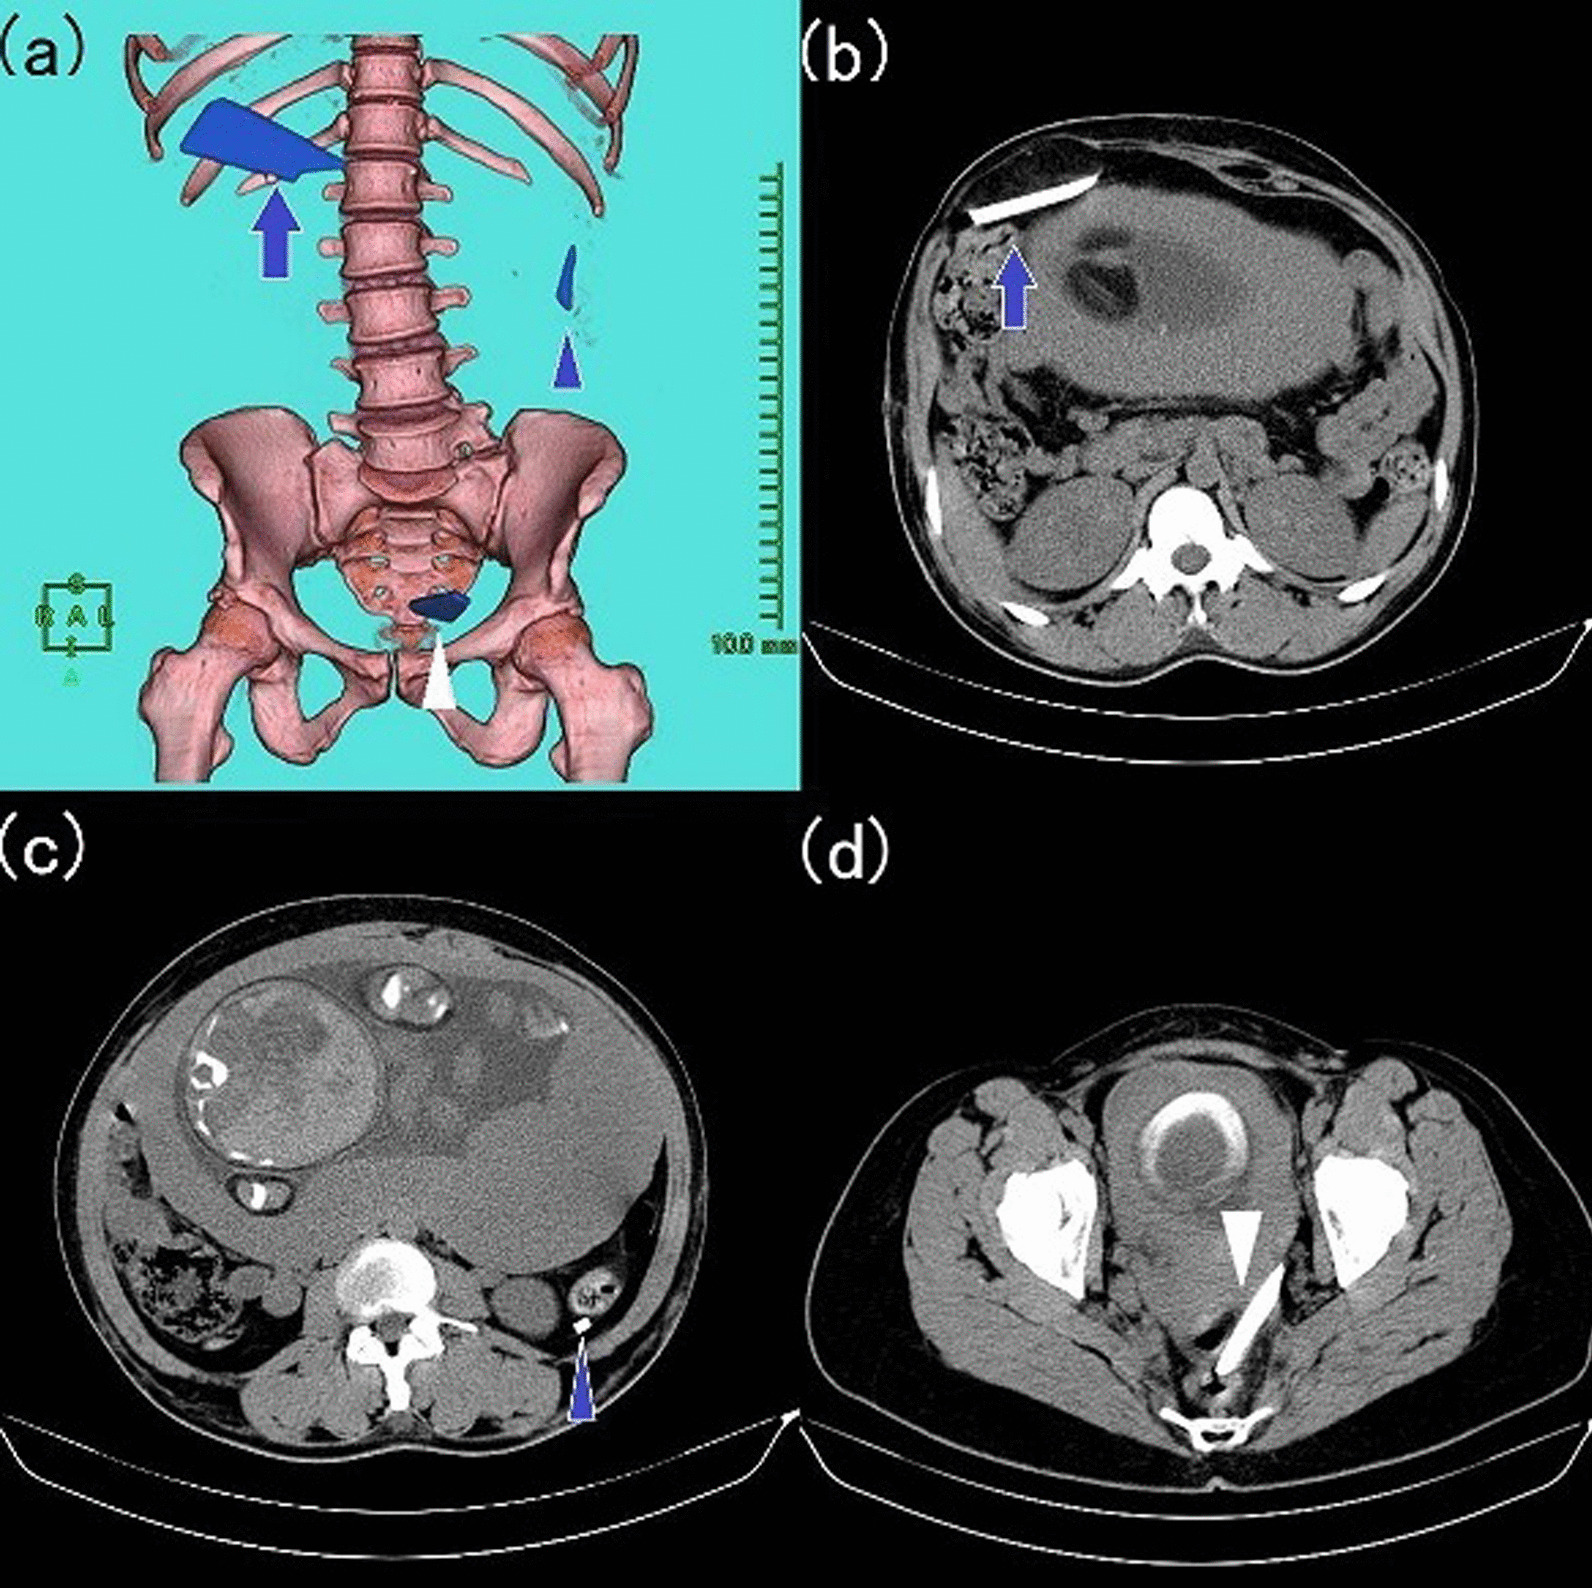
a Abdominopelvic three-dimensional CT and b-d axial CT images at 33 weeks gestation. The glass fragments are shown in blue on the images. There was no change in the positions of the shards in the right hypochondrium (arrow) or near the dorsal side of the descending colon (arrowhead), but the CT scan of the pelvic cavity revealed another 6 cm shard (white arrowhead) in the pouch of Douglas
